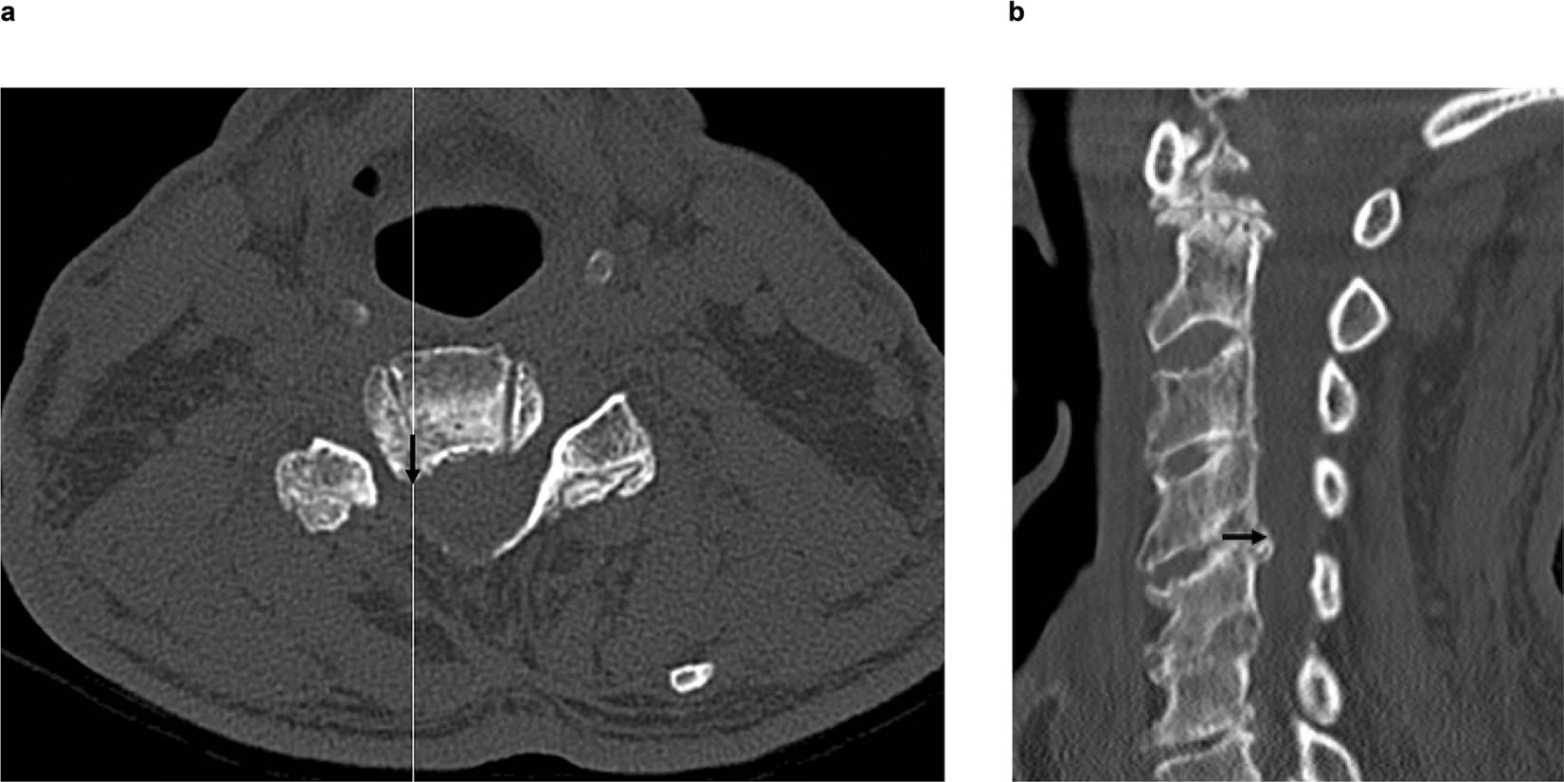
(a) Axial CT: Line is a sagittal slice direction; arrow is an osteophyte direction. (b) Sagittal CT: Arrow is an osteophyte direction. We can clearly recognize the bony spur in the parasagittal view.
